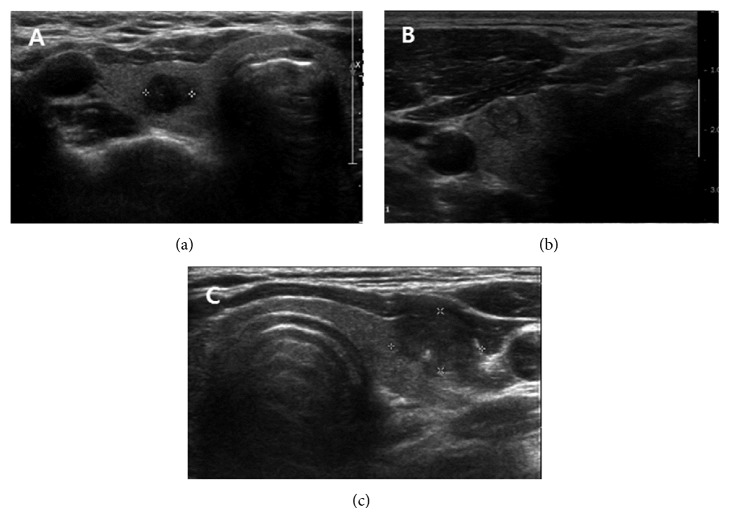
Grouping of thyroid tumor according to ultrasonographic findings. (a) Group 1: the tumor is completely enveloped by thyroid parenchyma, (b) Group 2: the tumor is attached to the thyroid capsule without definite destruction of the capsule, (c) Group 3: the tumor destroys the thyroid capsule and the capsule shadow is disconnected.
